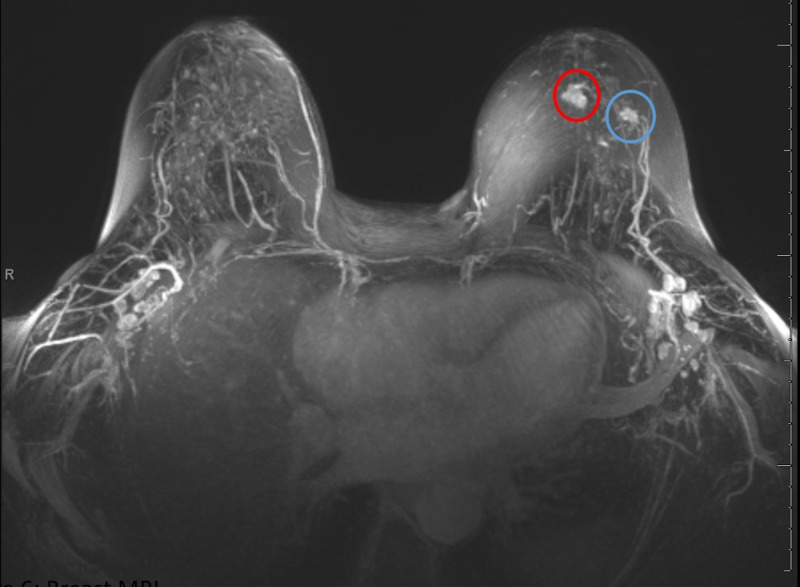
Breast MRI Left breast enhancing irregular masses corresponding with the mammographic sites of biopsy-proven invasive ductal carcinoma (IDC), measuring 15 mm at 12 o'clock subareolar region (red circle) and measuring 10 mm at 1 o'clock region, 5 cm from nipple (blue circle). There is no abnormal enhancement at the site of biopsy-proven ductal carcinoma in situ (DCIS) in the upper outer quadrant, 5 cm from nipple.
